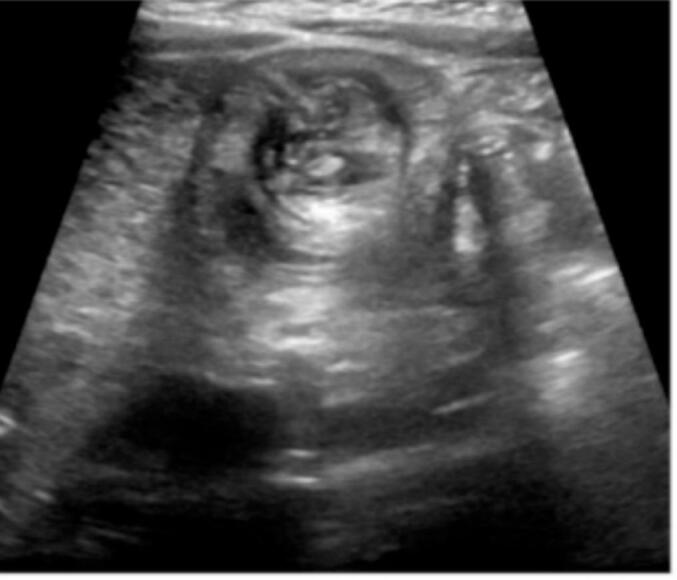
Abdominal ultrasound showed the classic “target sign” of intussusception at the ileocecal region.
